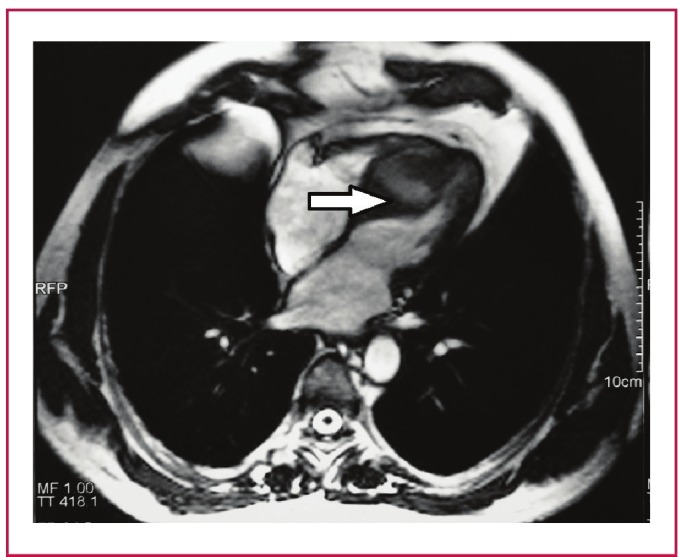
Magnetic resonance image showing the cystic mass in the interventricular septum (white arrow).
